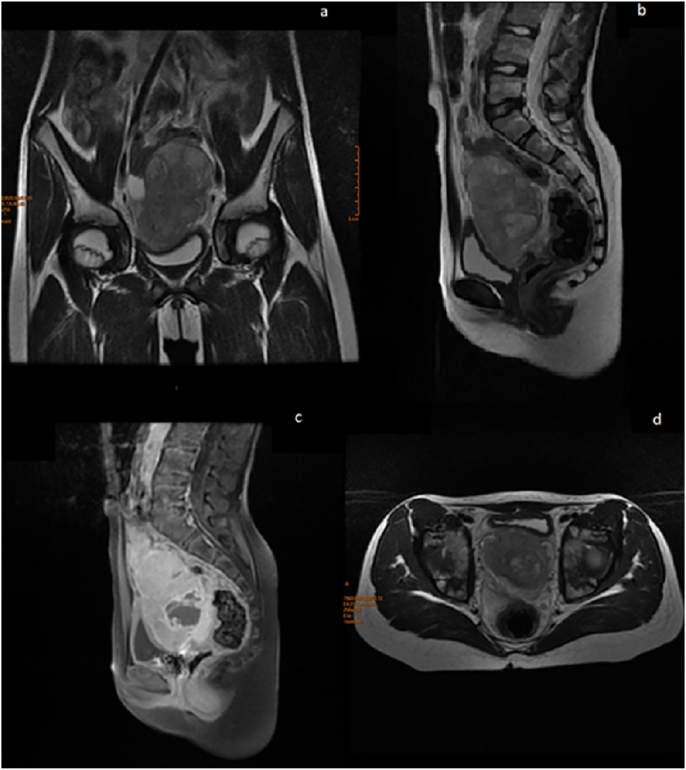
a. Coronal MRI view showing a right ovarian tumor with irregular seams. Peripheral hypersignal and central hyposignal T2 are in relation with a central necrosisb. Sagittal and d. Transverse MRI views showing bladder compression by the tumor. c. Sagittal MRI view showing a right ovarian tumor on hypersignal T1.
